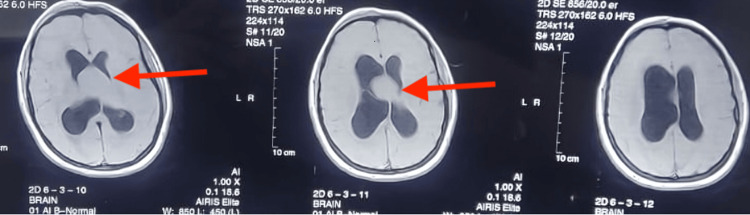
MRI images showing axial T1 sections of the brain There is a well-defined, hyperintense cystic lesion (red arrow) in the third ventricle with hydrocephalus
